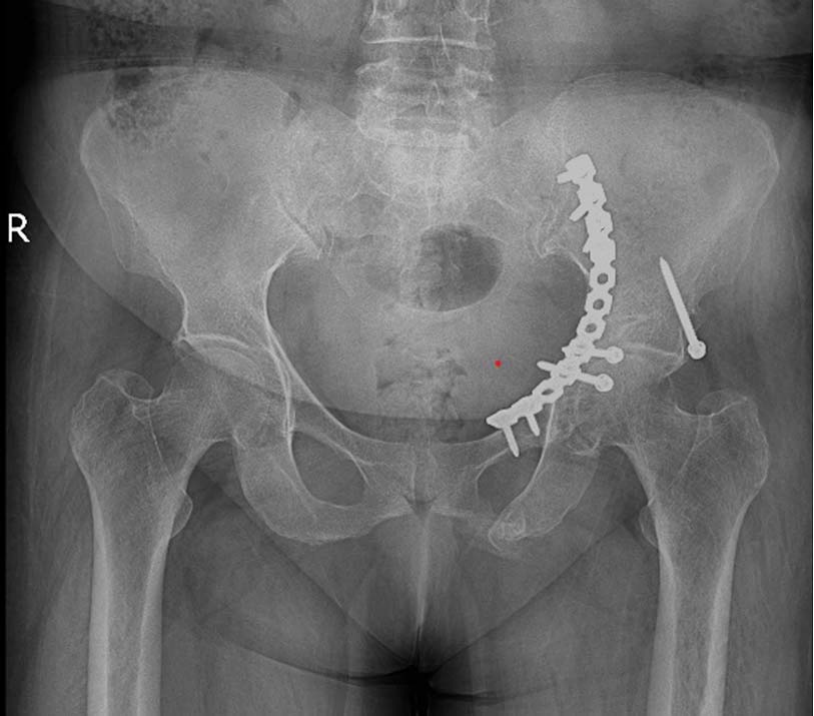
Plain pelvic radiograph after the surgery and fixation with plate and screws (anteroposterior view).
